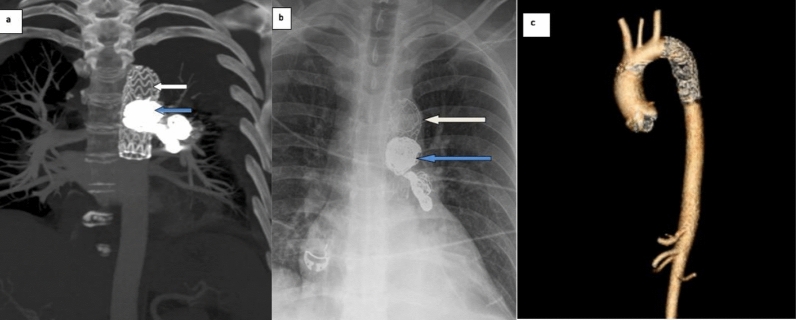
Post-intervention imaging demonstrating successful aneurysm exclusion following thoracic endovascular aortic repair (TEVAR) with adjunctive coil embolization. a Coronal multiplanar reconstruction CT image shows the endovascular stent graft within the thoracic aorta (white arrow) and embolization coils within the anomalous systemic-to-pulmonary vessel (blue arrow). b Chest radiograph demonstrates the thoracic endovascular stent graft and embolization coils. c Follow-up angiographic volume-rendered image shows absence of contrast opacification of the previously aneurysmal systemic-to-pulmonary vessel, consistent with successful treatment
